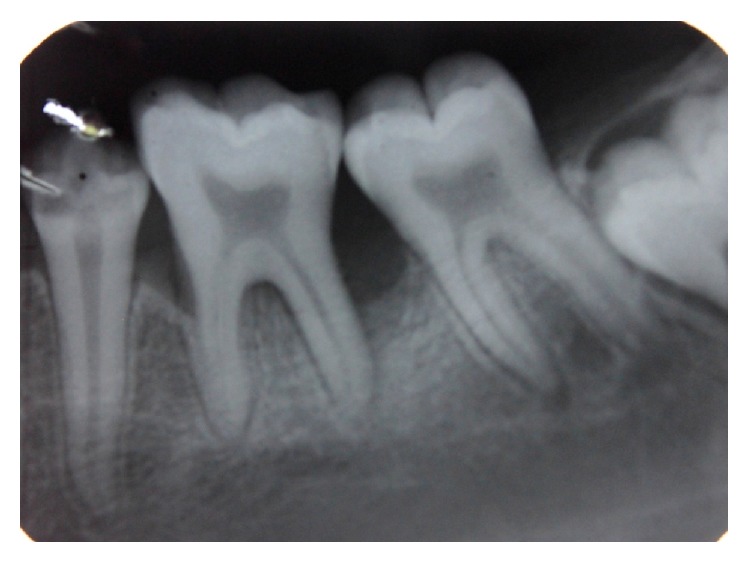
Intraoral periapical radiograph showing vertical bone loss in relation to 36.
